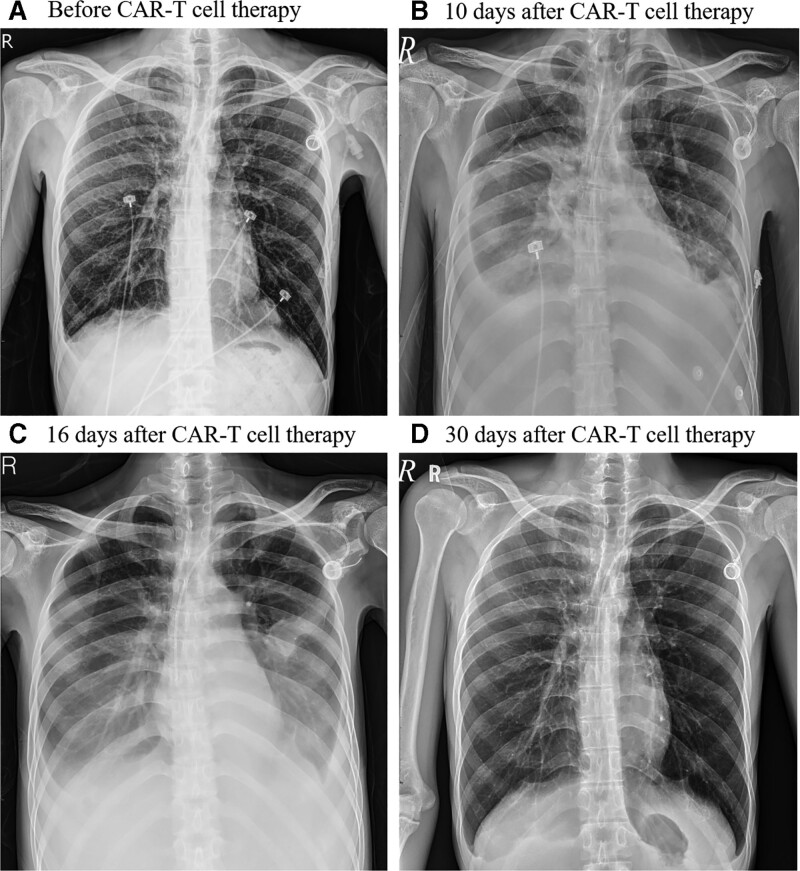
Serial chest plain films before and after CAR-T therapy. CAR = chimeric antigen receptor.
